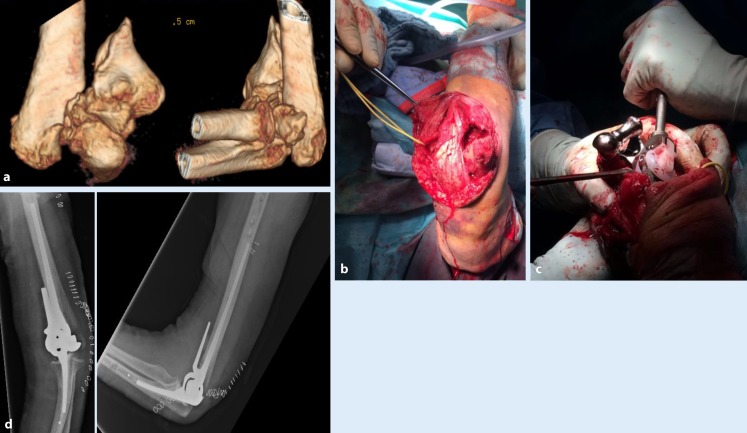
Nonreconstructable distal humeral fracture, AO 13 C3 (88-year-old female patient). a Preoperative computed tomography with three-dimensional reconstruction; b, c implantation of cemented linked total elbow arthroplasty using a triceps-on approach; d postoperative radiographs
